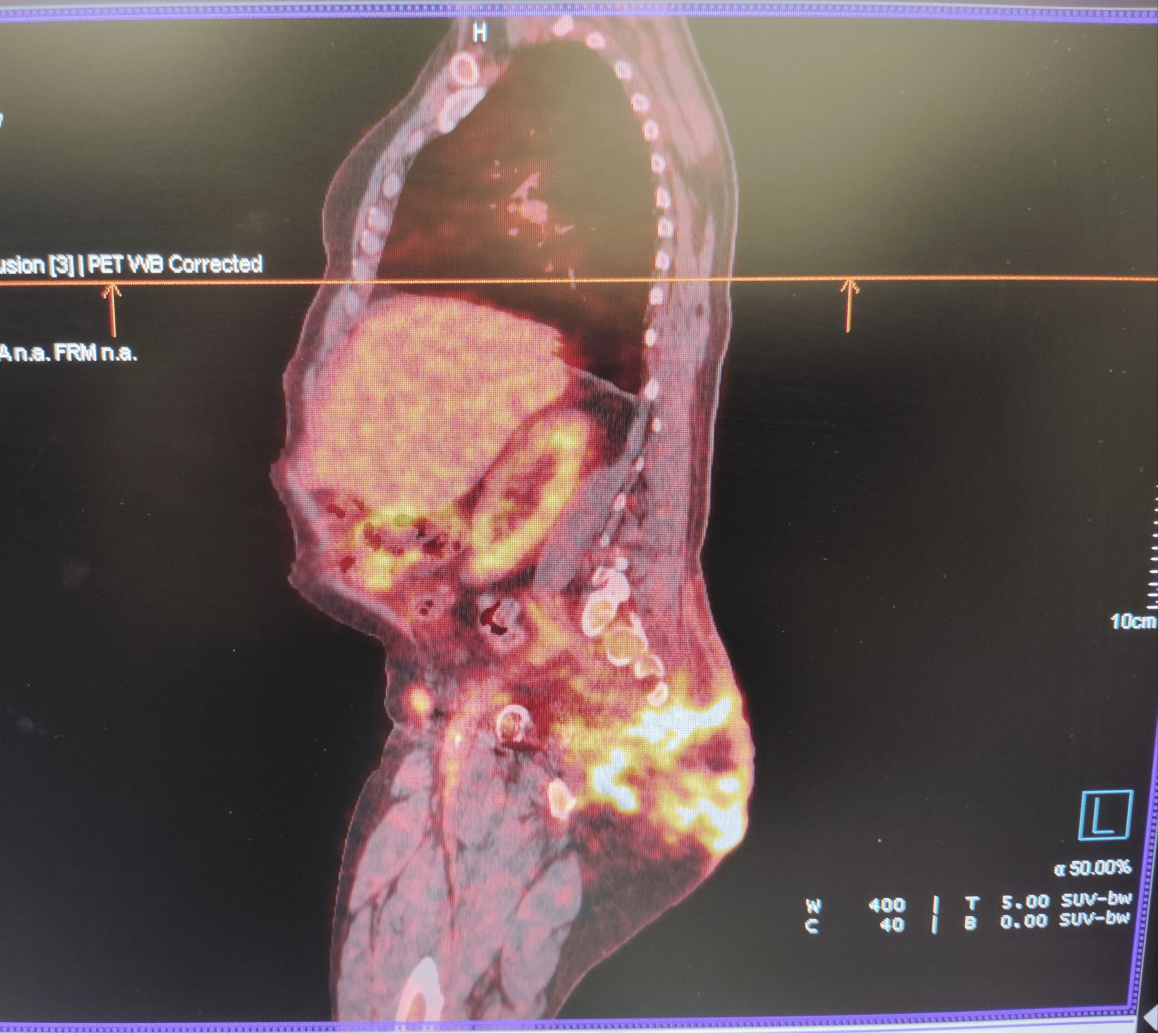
Baseline PET/CT scan performed in December 2023, prior to the initiation of cemiplimab and denosumab, showing extensive hypermetabolic tumor infiltration in the gluteal region and sacrum.
